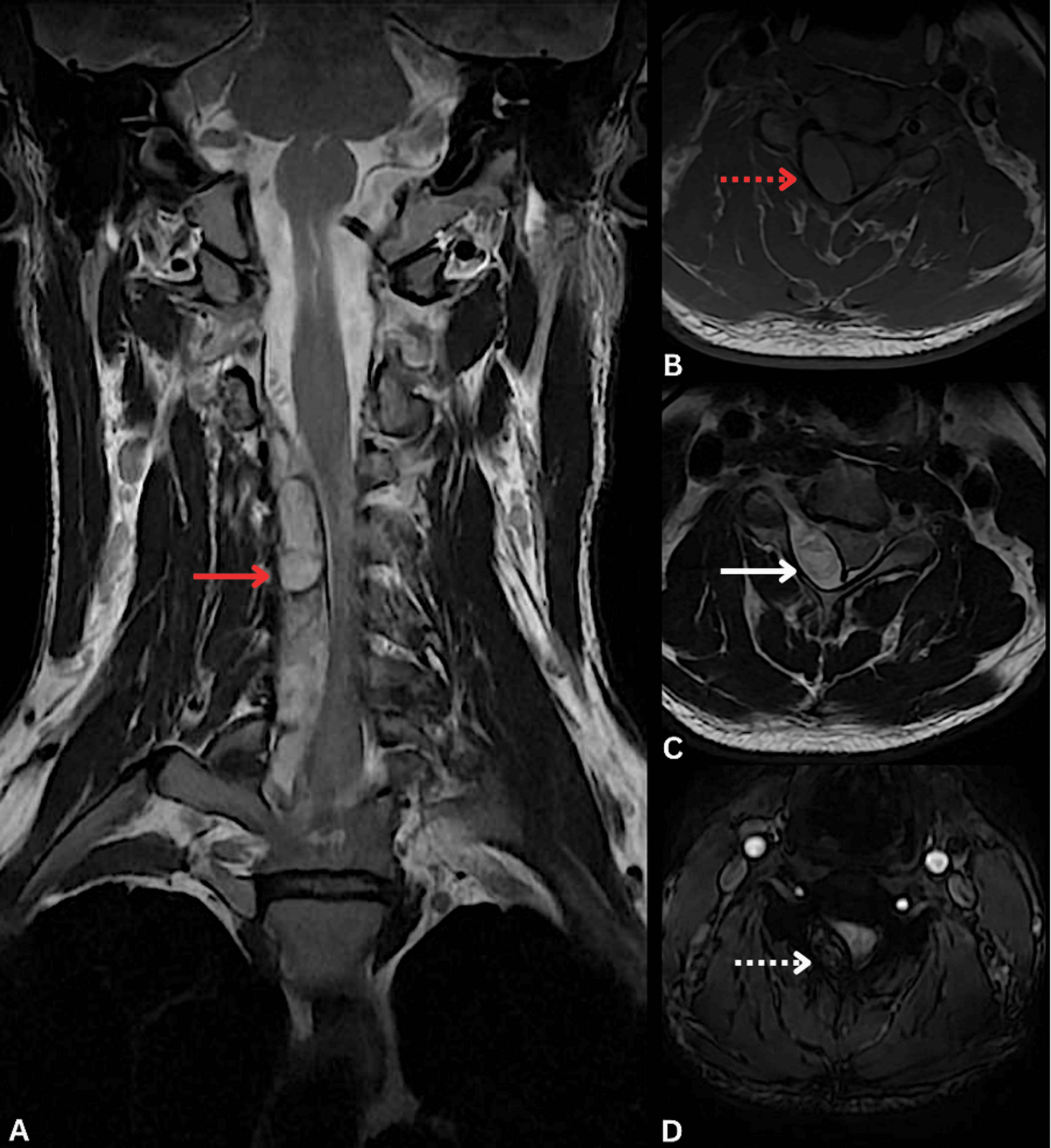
Contrast-enhanced cervical MRI showing an epidural hematoma extending from C3 to T2, causing spinal cord compression. (A) Coronal T2-weighted image: hyperintense epidural lesion (solid red arrow). (B) Axial T1-weighted image: isointense signal (dashed red arrow). (C) Axial T2-weighted image: hyperintense signal (solid white arrow). (D) Axial susceptibility-weighted image (SWI): peripheral hypointense rim with central hypointensity (dashed white arrow).
